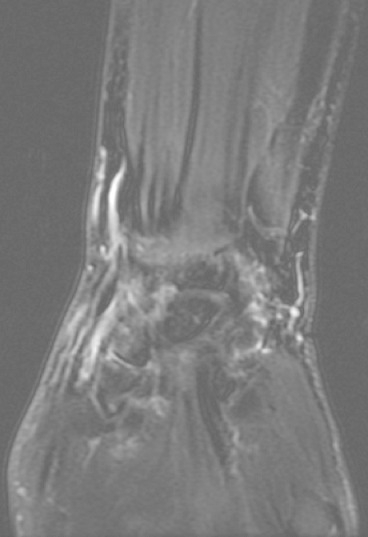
MRI coronal view showing view showing the extent of tenosynovitis
